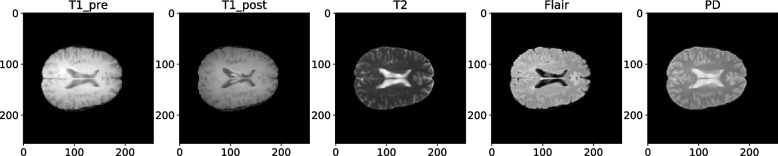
The MRI sequences of a patient as an example
